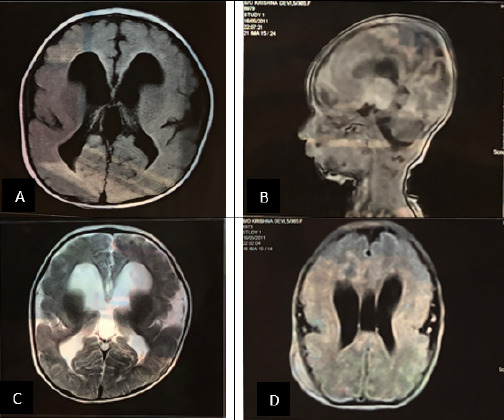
MRI showing ventriculomegaly with smooth parenchyma (A: T1 axial view, B: sagittal view, C: T2 axial, reduced sulci and gyri with smoothness, D: Axial/flair with cavum septum pellucidum/vergae).
